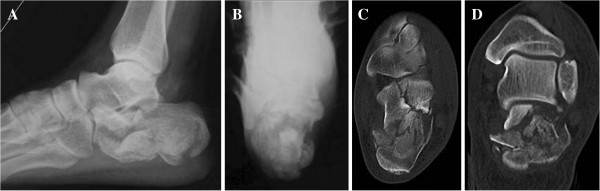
A 53-year-old male patient suffered a left bony destructive injury of the calcaneus due to a fall from a height. Preoperative radiographs (A, lateral view; B, axial view) and computed tomography (CT) scans (C and D, the sagittal images) demonstrate significant widening and shortening, severe loss of height of the calcaneus and collapsed articular surfaces.
